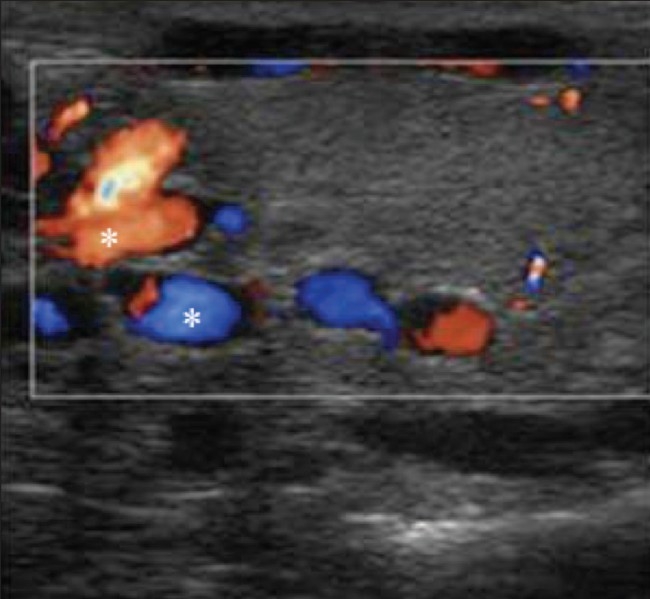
Varicoceles Color Doppler shows a venous pattern of color flow in the anechoic channels (asterix) confirming the diagnosis of both intratesticular and extratesticular varicoceles
